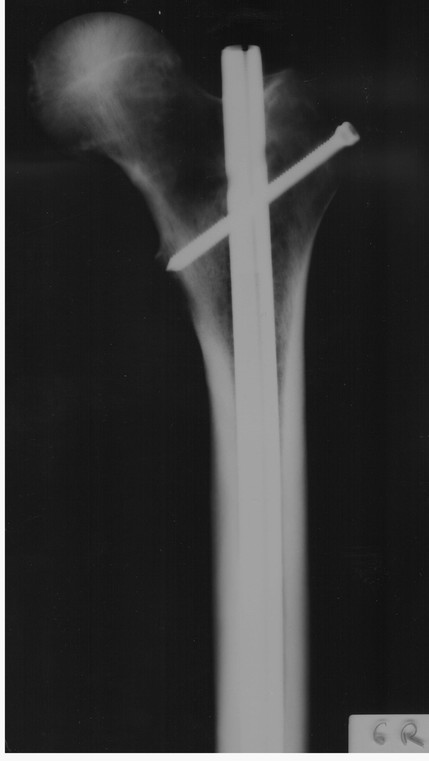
Anteroposterior radiograph of the same femur. No evidence of fracture line can be documented.
